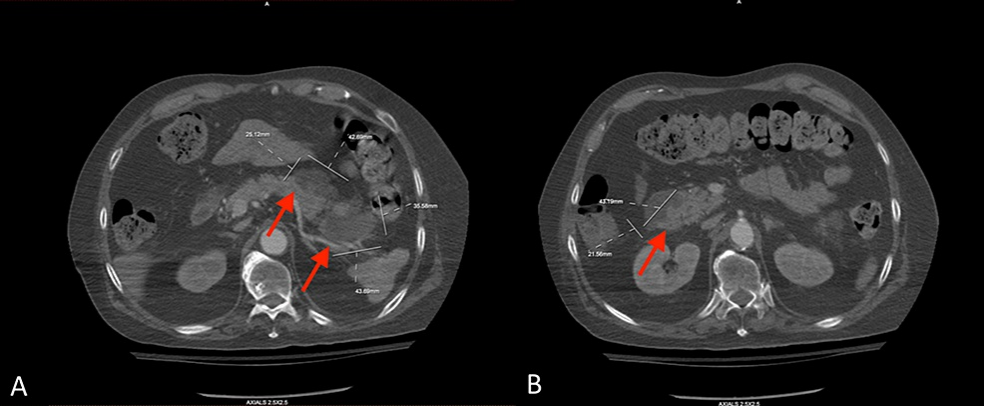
CT with IV contrast of chest A) CT with IV contrast of chest depicting lobular cystic masses visualized at the pancreatic head measuring about 4.3x2.2 cm in transaxial dimension, at the pancreatic body measuring about 2.5x4.3 cm, and B) in the pancreatic tail region measuring 4.4x3.6 cm (arrows).
